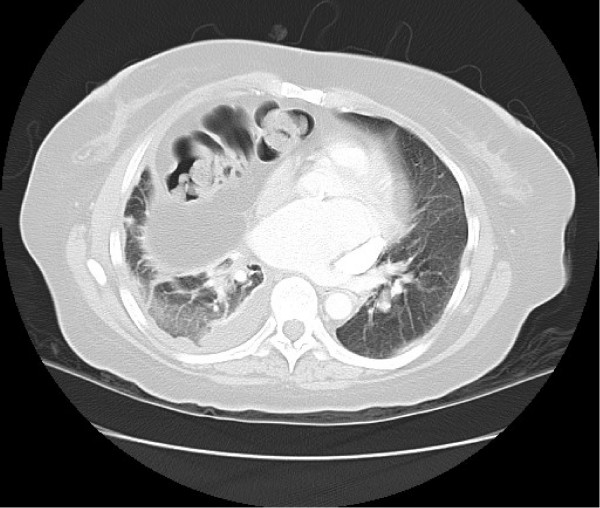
Axial computed tomography scan of the chest and abdomen demonstrating plate atelectasis in the right upper lobe presumably as a consequence of the longstanding right-sided anterior diaphragmatic hernia of Morgagni which displaces the heart to the left.
